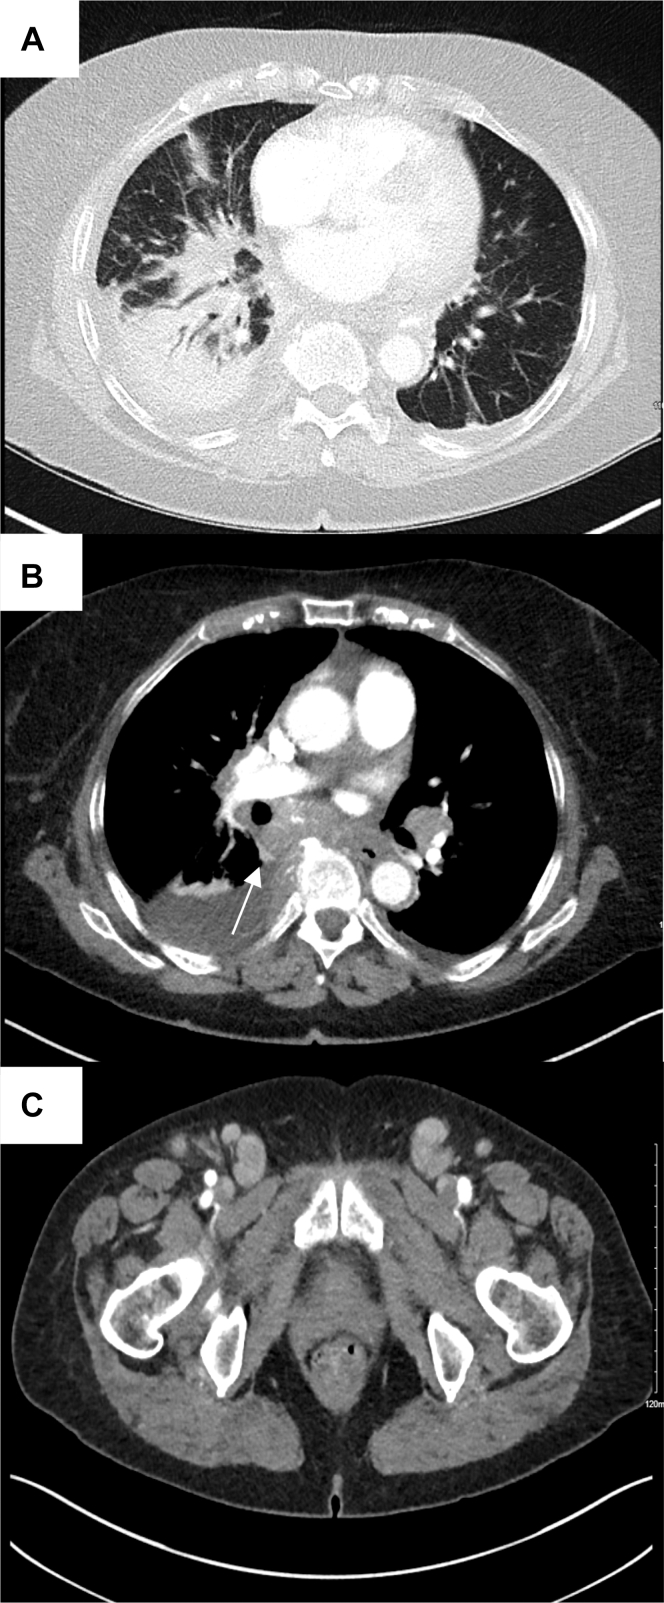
A, Axial CT thorax, abdomen, and pelvis lung windows showing a large partially loculated pleural effusion with extensive pleural nodularity and interlobular septal thickening along with diffuse pulmonary nodularity. B, Axial soft tissue windows from the same CT scan showing infiltrating mass around the right hilum with partially calcified hilar, subcarinal, and paraoesophageal lymph nodes (white arrow). C, Axial soft tissue window from the same CT scan showing bulky inguinal lymphadenopathy with the largest on the left 2.8 cm.
